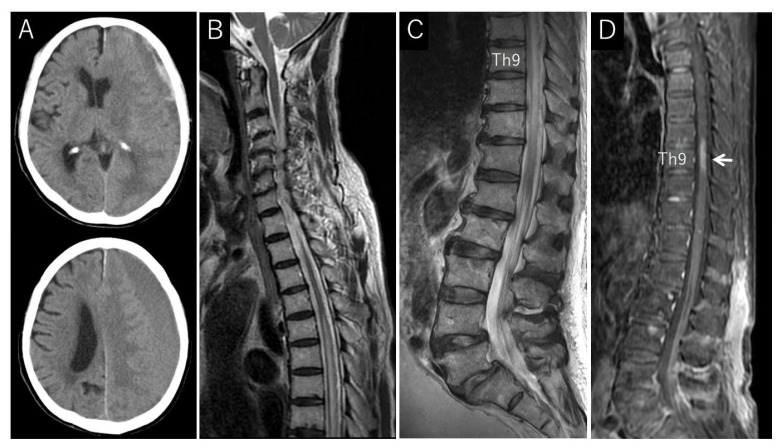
Computerized tomography (CT) and magnetic resonance (MR) images from a patient with dural arteriovenous fistula (DAVF); (A) CT scan of the head showing left chronic subdural hematoma; (B) sagittal T2-weighted MR image of cervicothoracic spine showing cervical canal stenosis, spinal cord edema, and flow voids around the cord; (C) sagittal T2-weighted MR image of thoracolumbar spine, highlighting spinal cord edema and flow voids around the cord; (D) sagittal contrast-enhanced MR image displaying enhancement of spinal cord condition (arrow).
